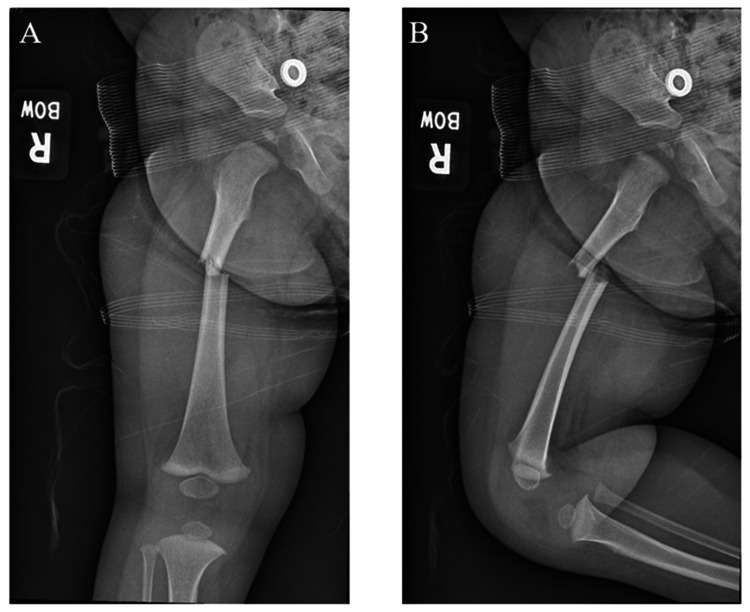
Initial imaging for case 1, including (A) AP and (B) lateral radiographs of the right lower extremity demonstrating a transverse fracture of the proximal third of the femur
